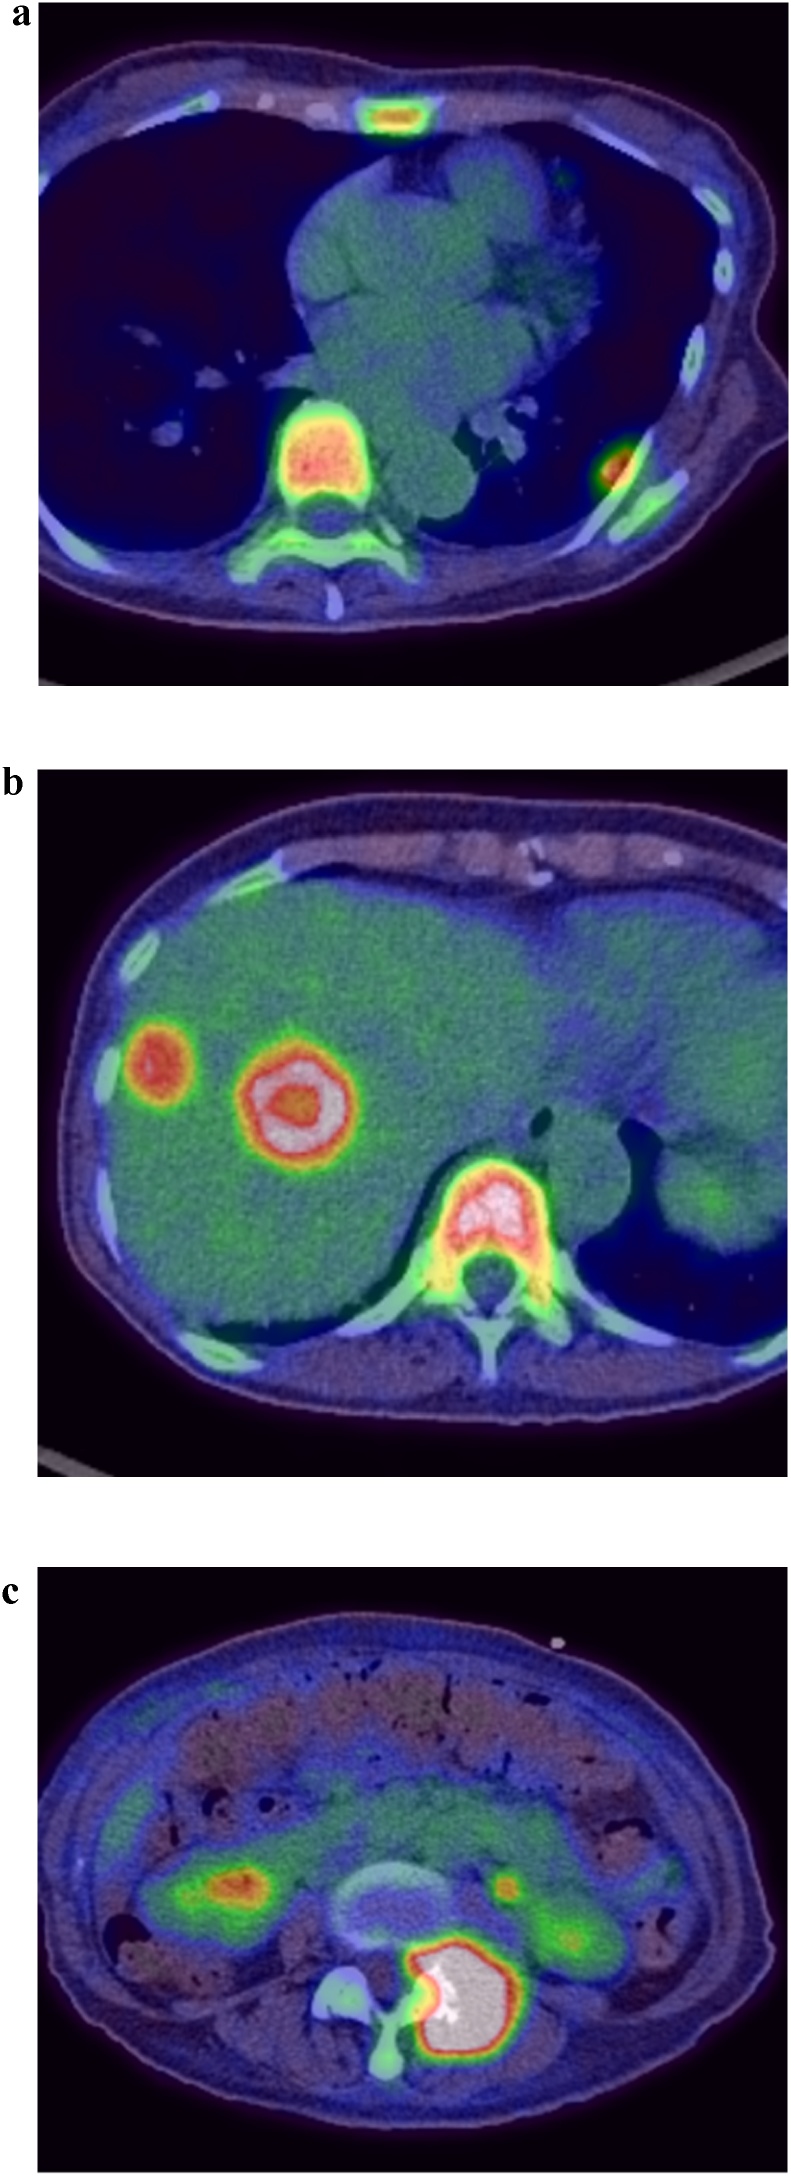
a–c PET findings after surgery. PET demonstrated an accumulation of FDG in the pelvic, lung, liver and vertebral body.
